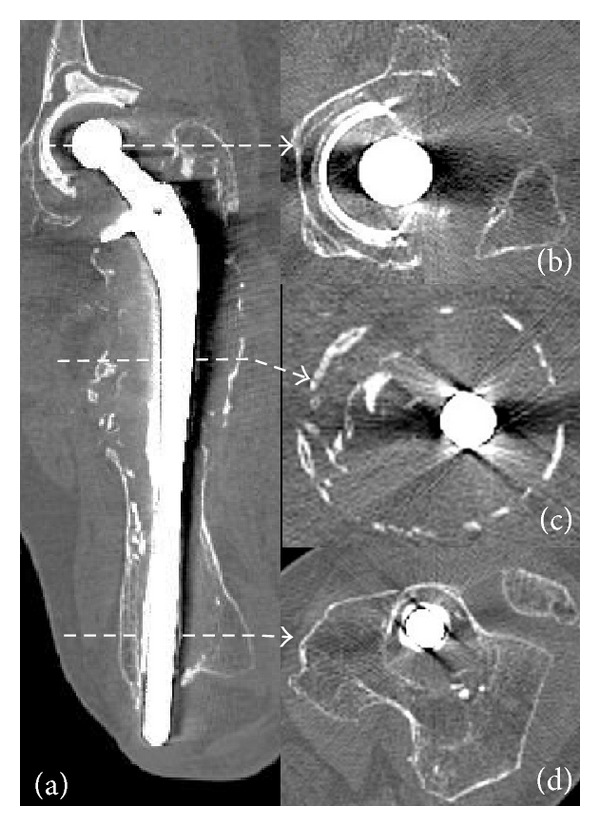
Computed tomography (CT) images of the left femur. Coronal CT image of the left femur shows a massive osteolytic lesion involving two-thirds of the proximal femur with thin cortical fragments surrounding the stem (a). Most of the cement mantle has disappeared except around the greater trochanter and middle of the stem. Axial views from the dotted lines are noted (b)–(d).
